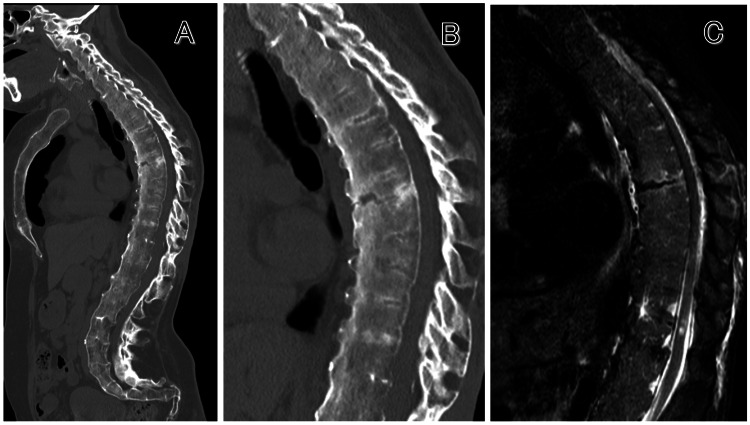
Preoperative images. (A, B) Computed tomography scan showing ankylosis of the entire spine and reverse chance-type sixth thoracic vertebral fracture. (C) Magnetic resonance image showing no obvious damage to the posterior ligament complex.
